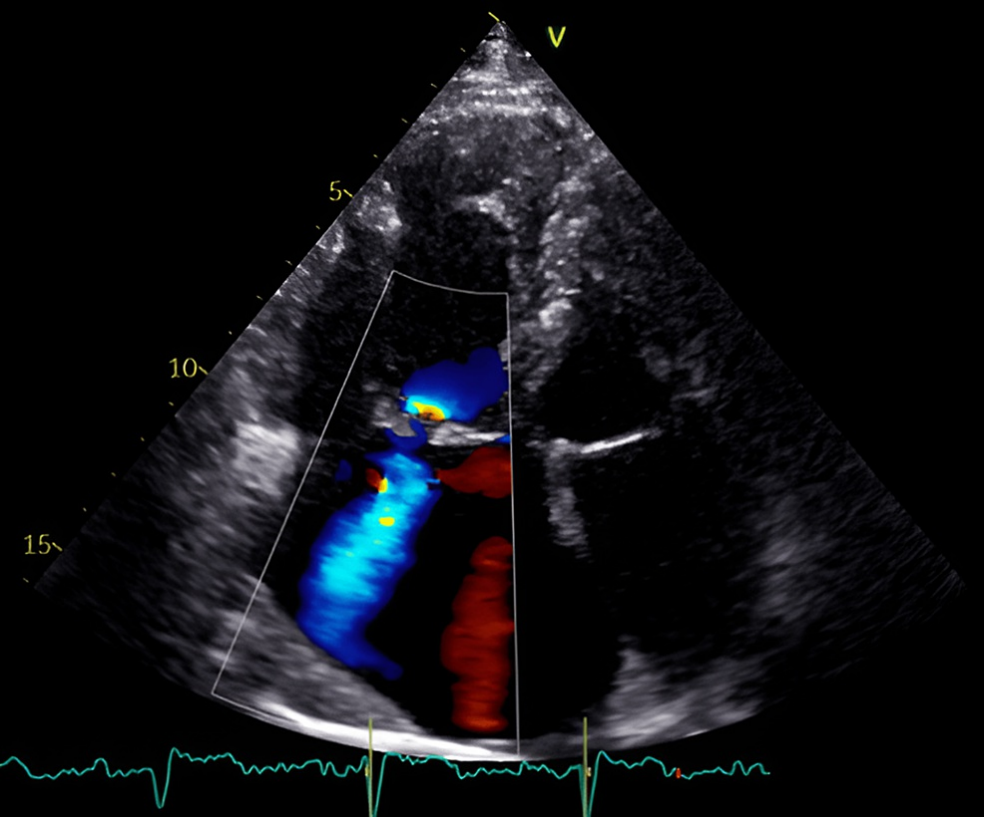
TTE (apical four-chamber view) showing moderate tricuspid regurgitation, significant dilatation of right heart chambers and left atrium TTE: transthoracic echocardiography
